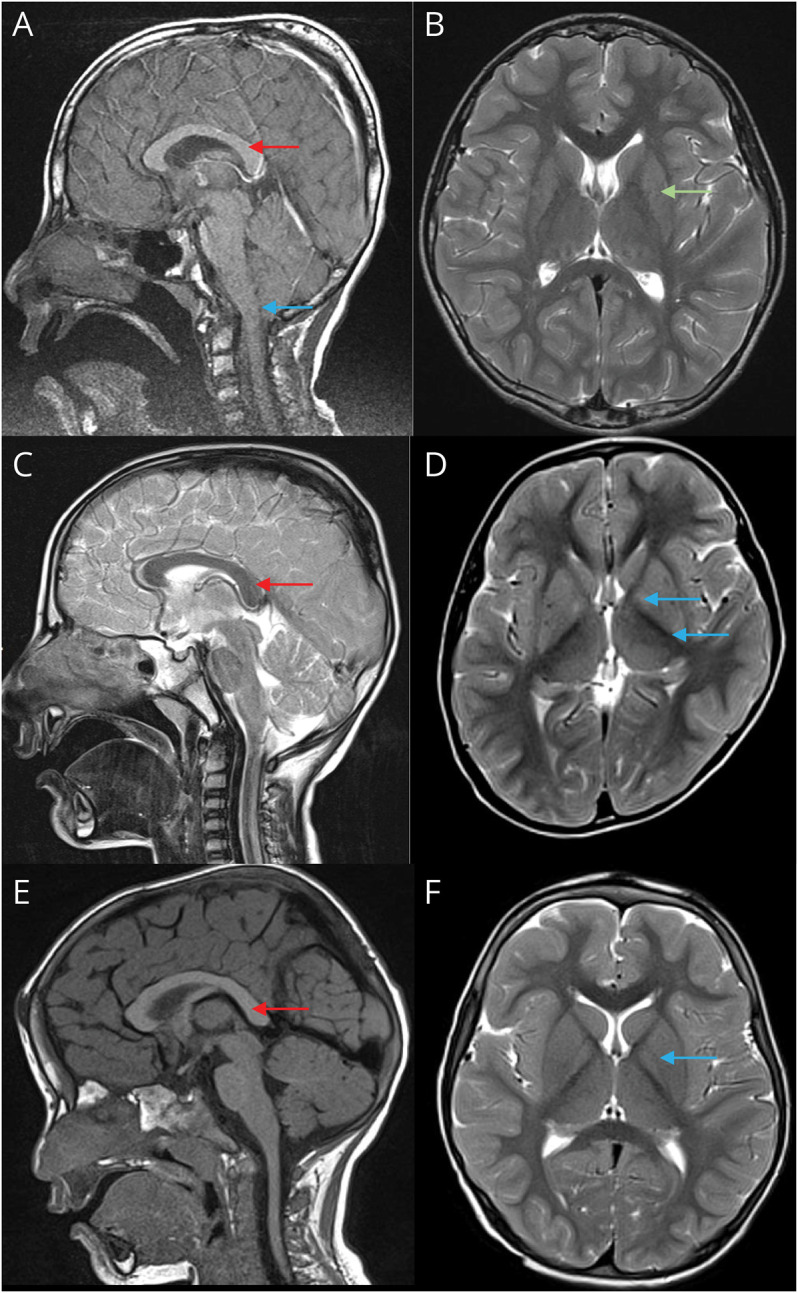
MRIs From Participants (A) F1(II-2) sagittal, T1 weighted, showing dysmorphic corpus callosum (red arrow) and shallow pontomedullary sulcus (blue arrow). (B) F1(II-2): axial, T2 weighted, showing small-volume basal ganglia (green arrow). (C) F2 sagittal, T2-weighted, showing vertical posterior corpus callosum (red arrow). (D) F2 axial, T2 weighted, showing symmetrical hyperintensity of the globus pallidus and posterior putamen (blue arrows). (E) F3(II-5) sagittal, T1-weighted, showing vertical posterior corpus callosum (red arrow). (F) F3(II-5) axial, T2-weighted, showing small globus pallidus with altered signal intensity (blue arrow).
